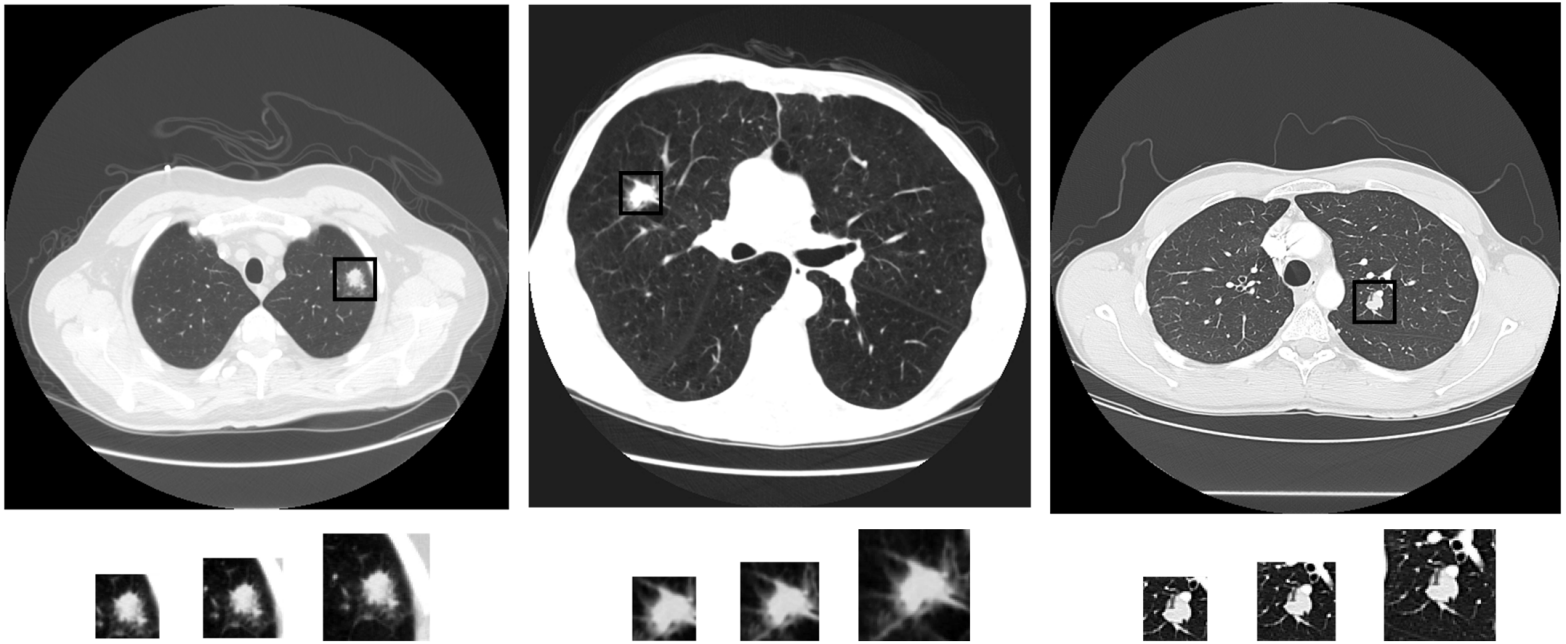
CT examples with lung nodules in different categories. They are benign (left), primary malignant (middle) and metastatic malignant (right), alone with 3 different view areas including 40×40, 50×50 and 60×60.
